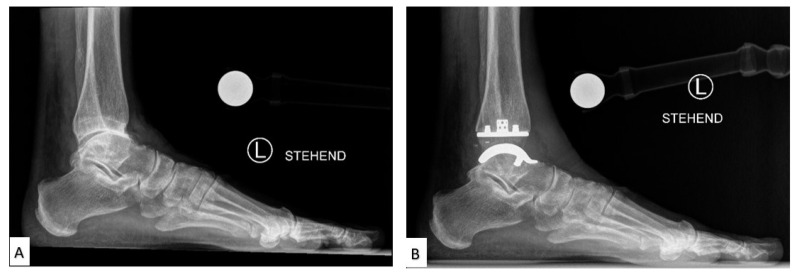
Labeled X-ray of (A) preoperative and (B) TAA implant performed on a patient, consisting of a polyethylene tibial component and metallic talar component [26].
